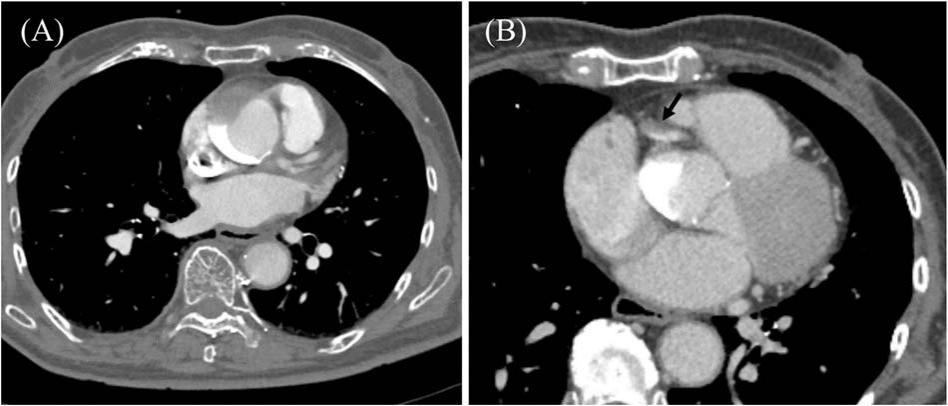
Preoperative contrast-enhanced CT. (A) Ascending aortic dissection is detected. Contrast-material is pooled in the false lumen. (B) Dissection at the proximal part of RCA is suspected (black arrow).
